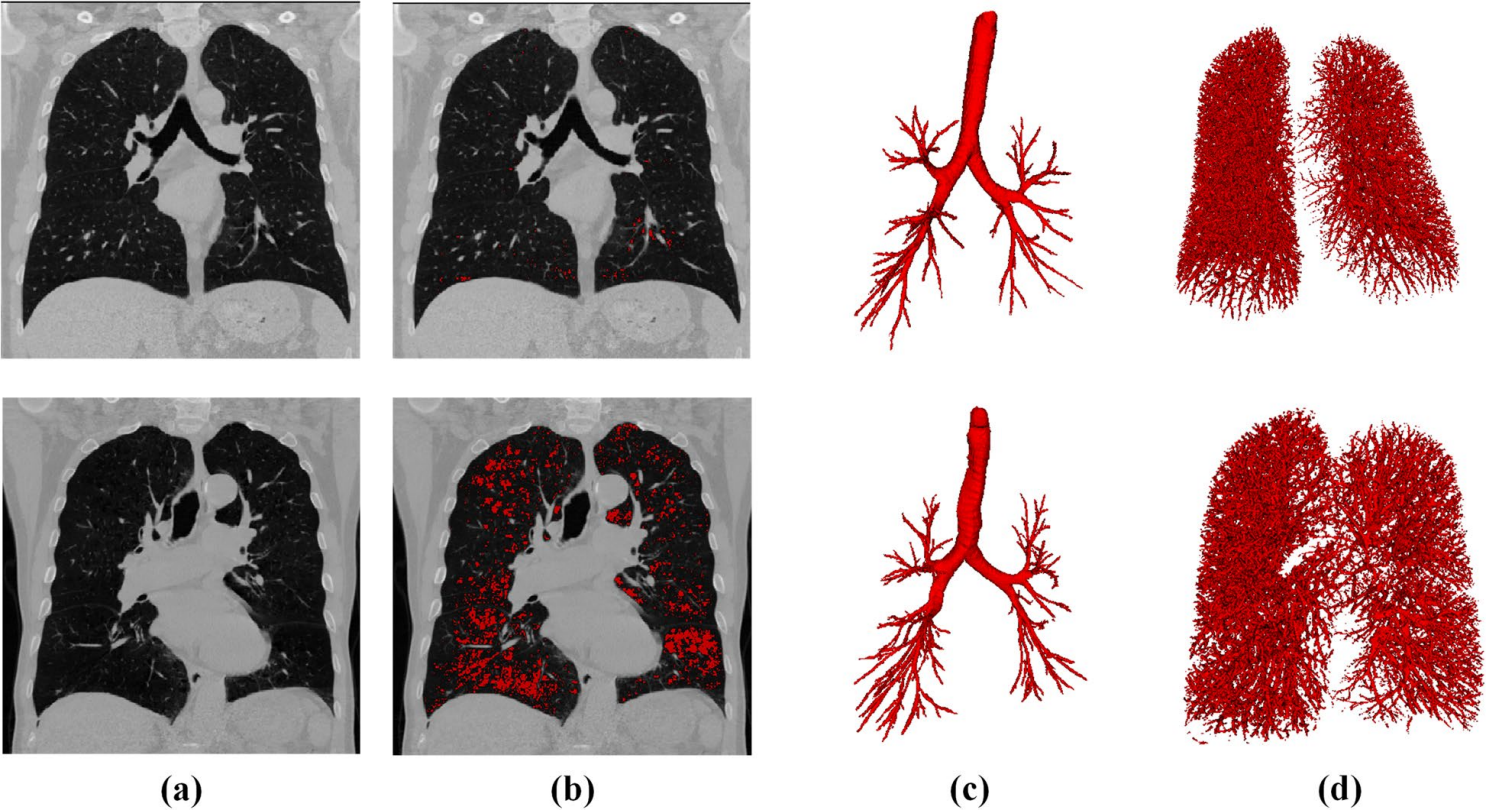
Coronal computed tomography (CT) view, emphysema region(in red), airway and vascular morphology in two COPD subjects with different stages. (Top) Male, Age = 51, BMI = 28.52, GOLD 1, FEV1 = 3.29, FVC = 4.75. (Bottom) Male, Age = 81, BMI = 25.49, GOLD 2, FEV1 = 1.71, FVC = 3.77. Emphysema index was calculated using a threshold of -950 HU in inspiratory CT scan [61]. The lung airway was extracted using the method in [59]. And lung vessel was segmented by [60]. BMI: body mass index; COPD: chronic obstructive pulmonary disease; FEV1: forced expiratory volume in one second; FVC: Forced Vital Capacity; GOLD: Global Initiative for Chronic Obstructive Lung Disease
